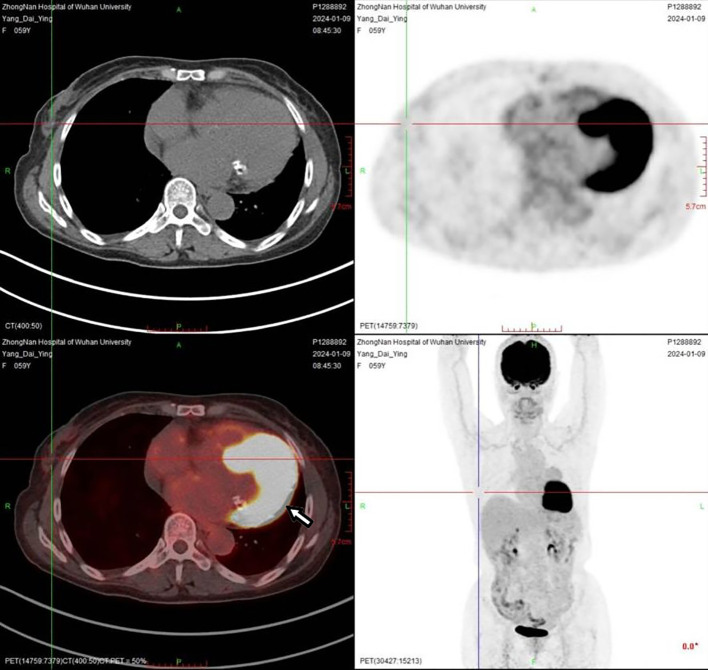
FDG-PET demonstrated heterogeneous fluorodeoxyglucose uptake within the tumor, suggestive of a malignant neoplasm.
